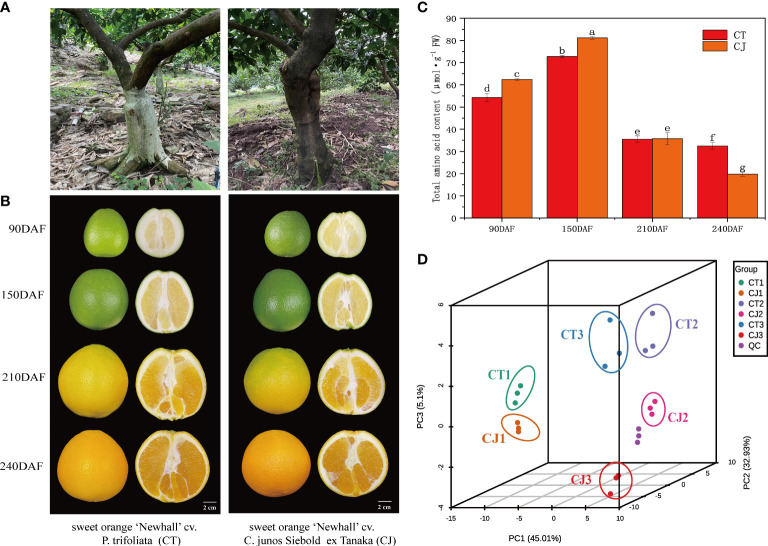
Appearance characteristics of sample material. (A) Two distinct appearance characteristics of rootstocks, P. trifoliata and *C. junos Siebold ex Tanaka*. (B) Pictures of CT and CJ peels in four different development periods. (C) Total amino acid content in CT and CJ peels. Red columns indicating the total amino acid content in CT; orange columns indicating the total amino acid content in CJ. (D) PCA analysis of amino acids in different samples.
